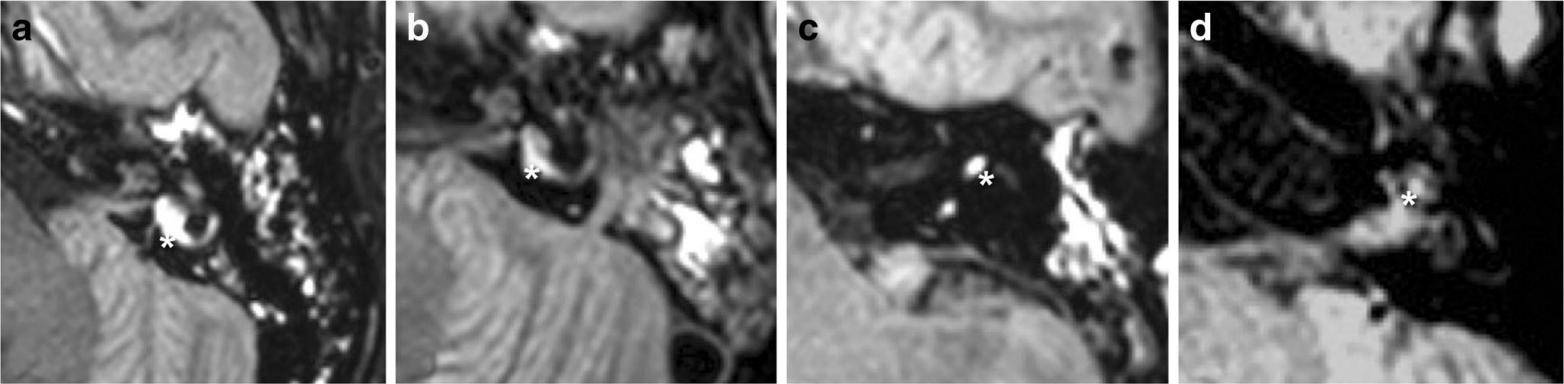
Examples of inner ear haemorrhage: the inner ear haemorrhage (*white star*) appears as a hypersignal on nonenhanced FLAIR acquired images of the vestibule (a and b), superior semicircular canal (c), and the cochlea (d). The first and third patient (a and c) referred with trans-labyrinthine fracture, the second (b) with extra-labyrinthine fracture, and the fourth (d) with major brain trauma, without temporal bone fracture. All patients presented post-traumatic SNHL, ipsilateral to the haemorrhage
